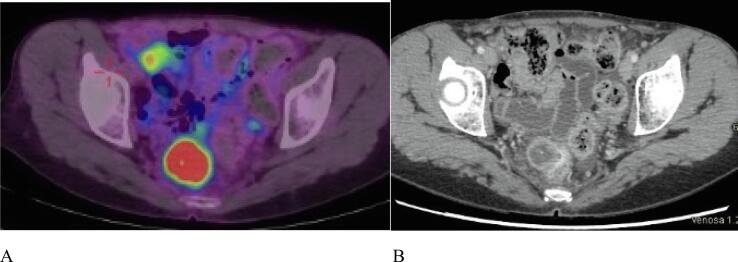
A: PET scan. B: CT scan, May 2022, showing a hypodense lesion (25 × 22 mm) located in the posterior mid-rectal wall.
